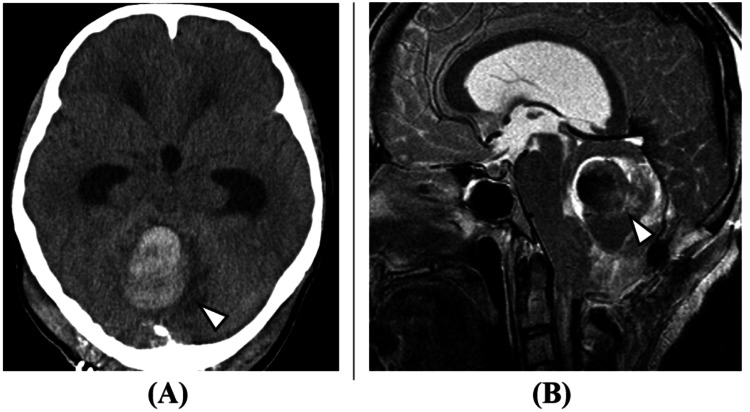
Brain imaging: (A) brain CT image (axial view) and (B) brain MRI image (T2 sagittal). Brain imaging studies conducted four days after neuroendovascular treatment show an increase in the size of the cerebellar hemorrhage, suggesting rebleeding (arrowheads in A and B).
